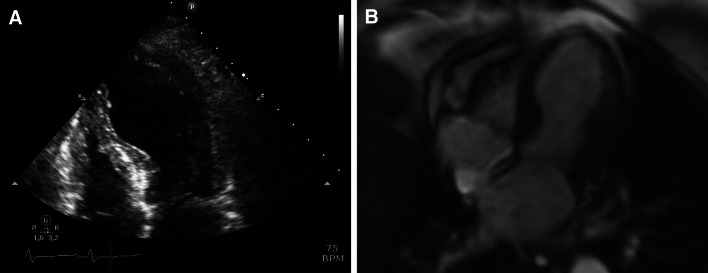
Examples of Tako-tsubo cardiomyopathy assessed with echocardiography (a) and MRI (b) showing typical apical ballooning with hyperkinesia of the basal segments and dyskinesia of the apical segments of the LV
